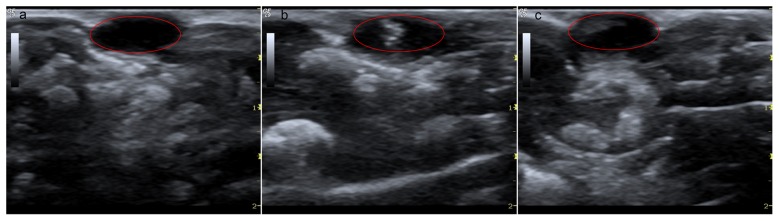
In vivo* B-mode ultrasound images of subcutaneous tumor with injection of DiR-SPIO-NDs. Ultrasound images were obtained with Logiq9 system with a 9L linear transducer at 8.0(a) Before the injection, (b) 1 min after injection, (c) 4.5 h after injection. The red circle denoted the echo enhancement in the tumor region.
